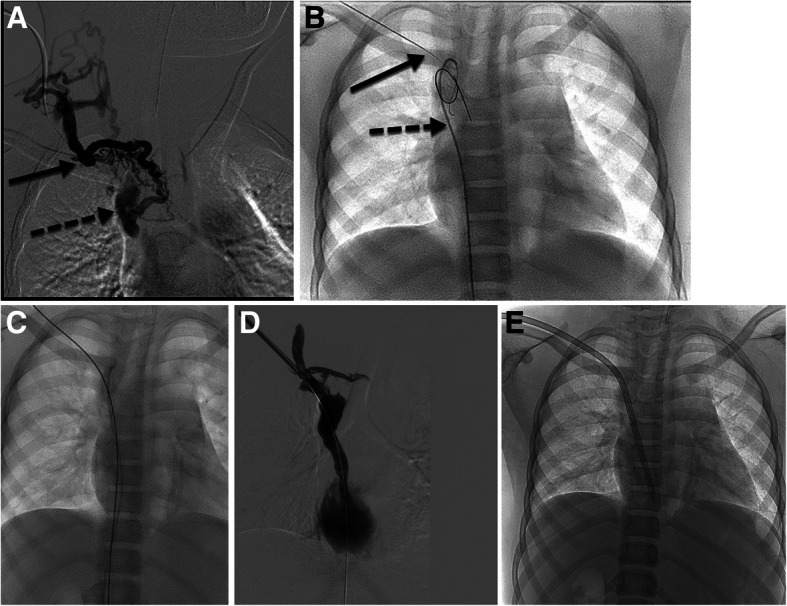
5 y/o M with end stage renal disease requiring hemodialysis with very limited central venous access (left internal jugular occluded and left femoral vein occluded). His hemodialysis catheter was inadvertently pulled out and central venous access lost. a Initial venogram from the right neck demonstrates numerous cervical collateral vessels (solid arrow) with no opacification of the right internal jugular vein. Collateral vessels eventually drain into the patent mid SVC and azygous vein (dashed arrow). b 22G 10cm Chiba needle passed behind the clavicle (solid arrow) part way with ultrasound guidance and residual guidance with fluoroscopy. Patent SVC target is marked with angled catheter and wire (dashed arrow). c Wire is then passed from the neck to the IVC and safety wire from the groin is retracted. Eventually the wire from the neck was snared and “flossed” access was achieved. d Post recanalization venogram demonstrates patent but slightly narrowed SVC with limited cervical collateral filling. e Hemodialysis catheter placement with tip terminating in the right atrium and tunnel created over the shoulder to reduce the risk of inadvertent catheter removal in the future
